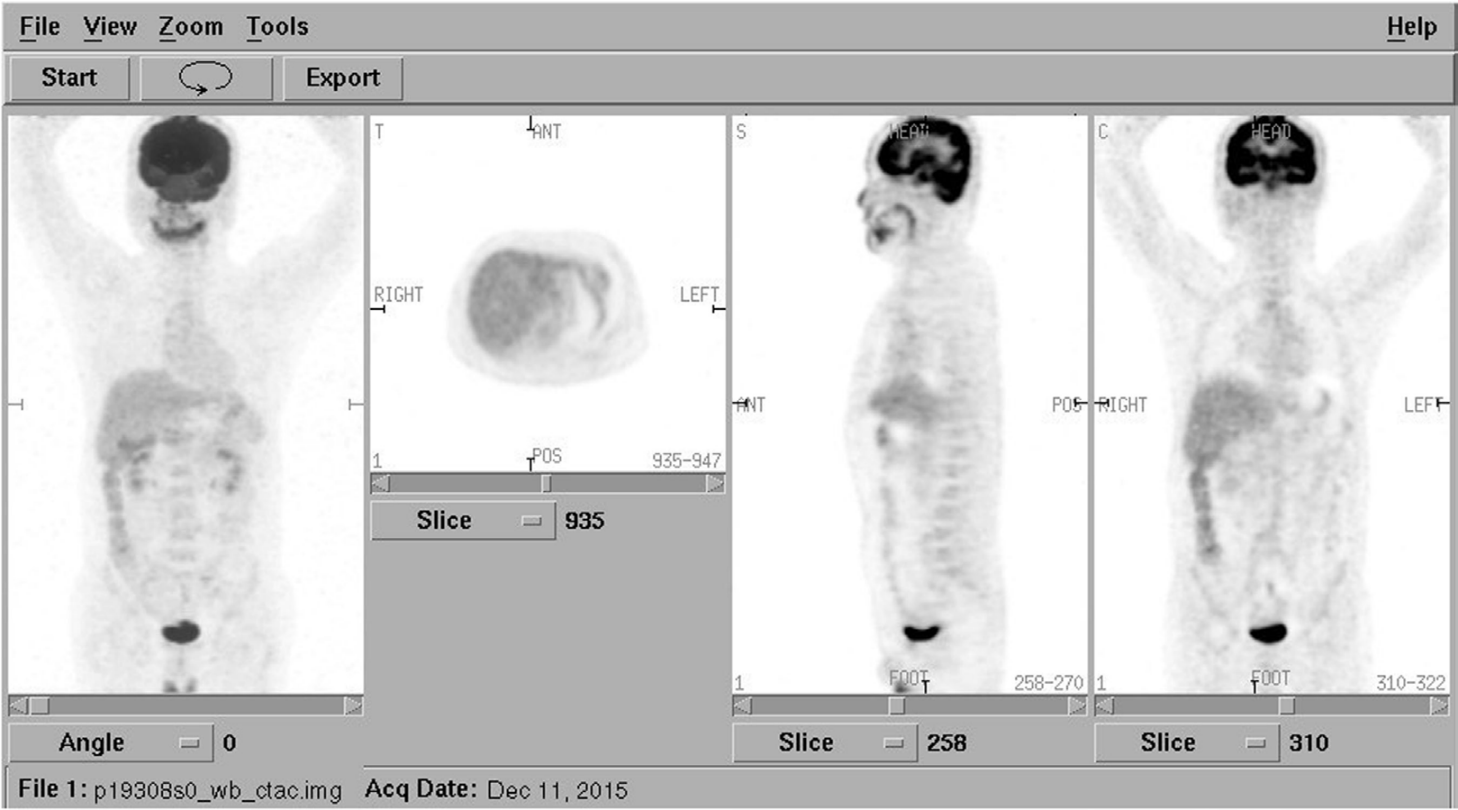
Positron emission tomography combined with computed tomography from the cerebellum to the upper thighs showing increased uptake in the mandibular bone body especially in the left mandible. No abnormal uptake in distant sites.
